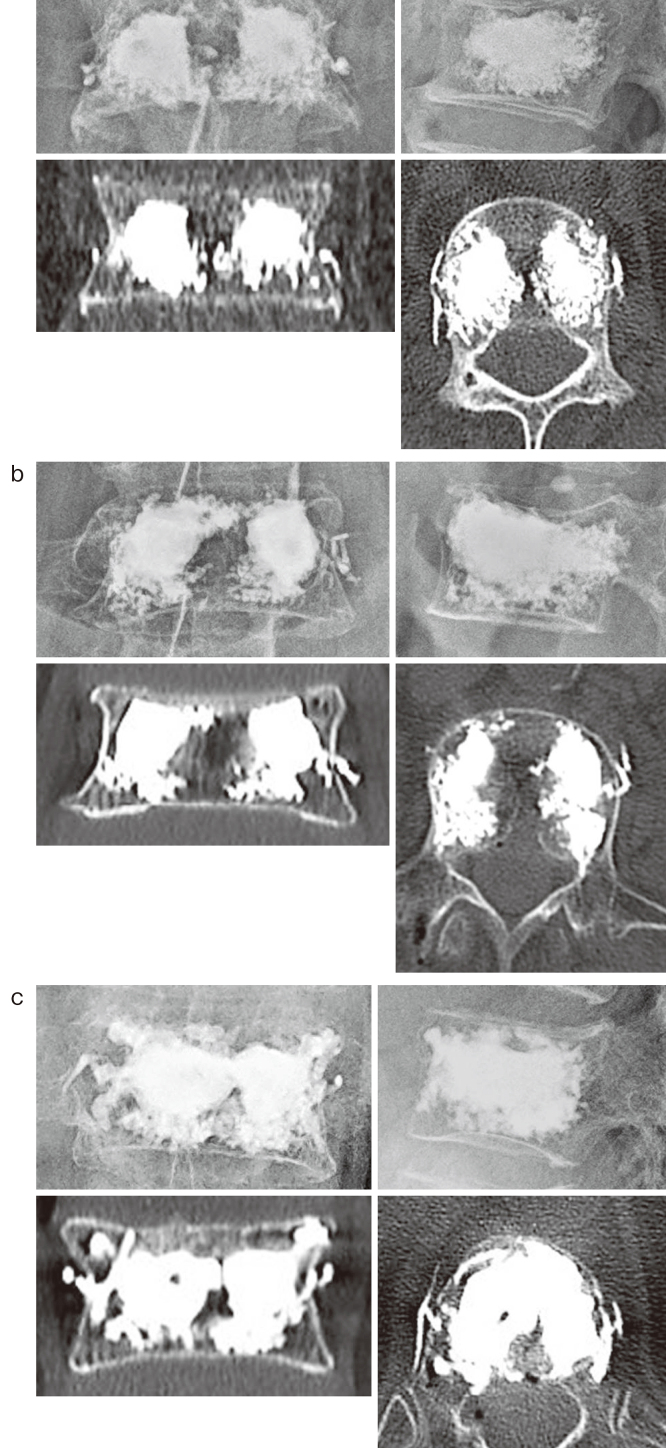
Postoperative X-rays from three cases who had cement embolization into the perivertebral veins. (a) Case 1. (b) Case 2. (c) Case 3.
